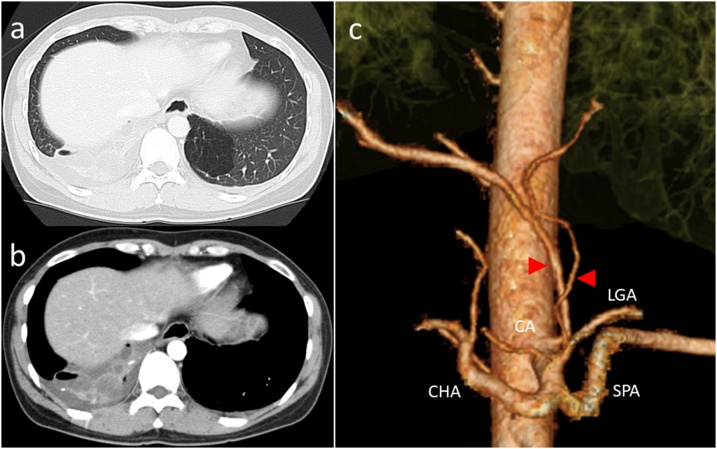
Chest computed tomography (CT) shows bilateral intralobar pulmonary sequestration. CA, Celiac artery; LGA, Left gastric artery; CHA, Common hepatic artery; SPA, Splenic artery; (a) Multiple cystic lesions in the right lower lobe were detected in the posterobasal segment of the right lung. (b) Hyperinflated lesion can be seen in the identical segment of the left lung. © Right aberrant artery arising from the celiac artery and left aberrant artery arising from the left gastric artery entering the affected segments (arrowhead).
